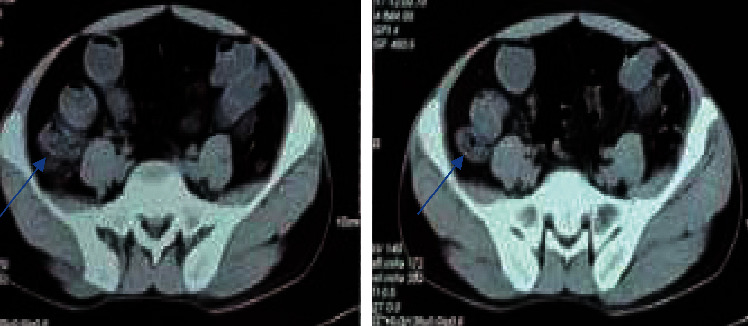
CT of the abdomen showing asymmetric circumferential thickening in the ileocaecal region with lobulated thickened caecum, soft-tissue stranding, and necrotic mesenteric lymphadenopathy.
